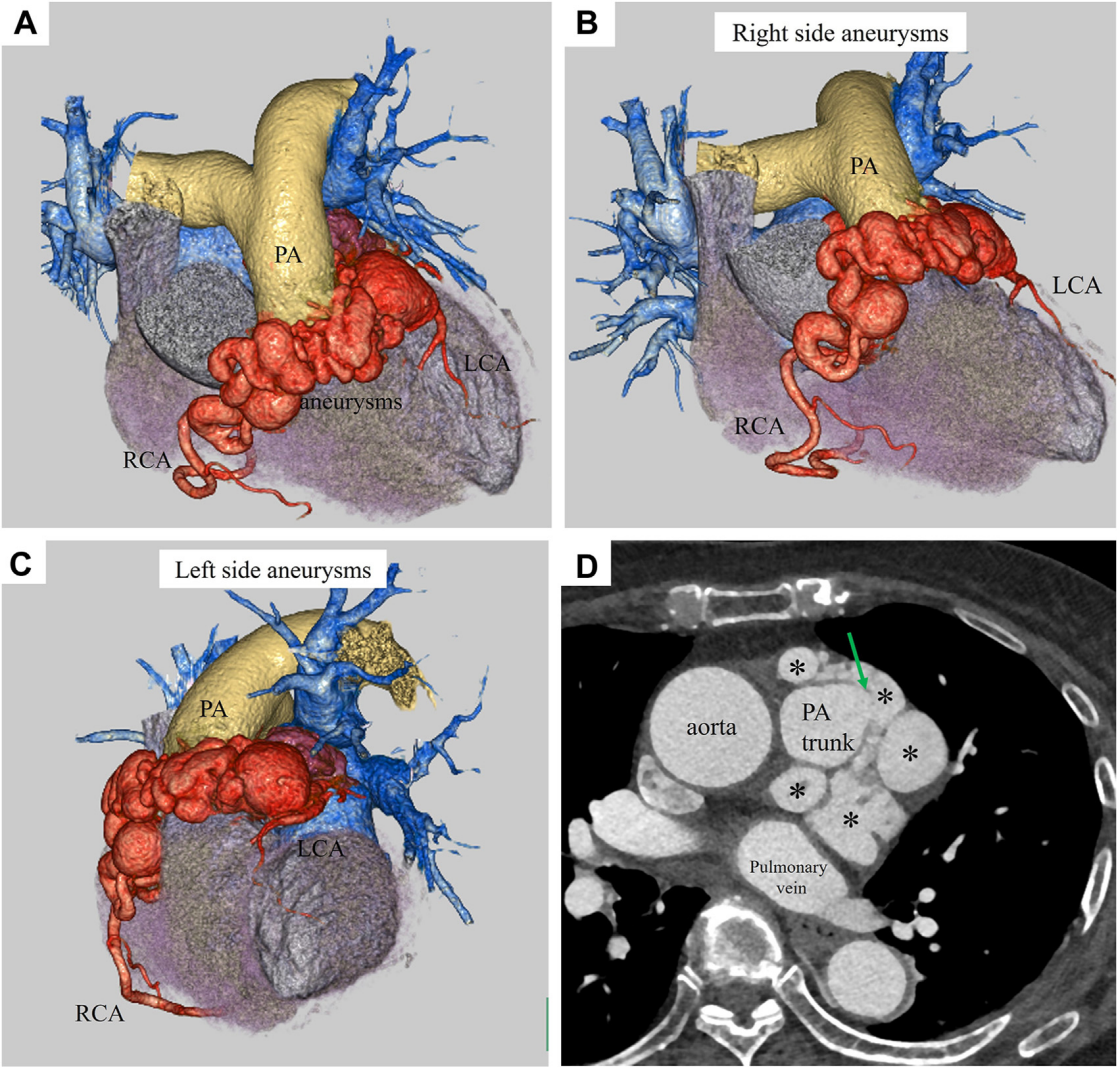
Preoperative multidetector-row computed tomography shows beaded giant coronary artery aneurysms (GCAA) in front of the pulmonary artery (PA) trunk. GCAAs (asterisk) connect the left coronary artery (LCA) with the right coronary artery (RCA). A GCAA-PA fistula is evident (green arrow).
